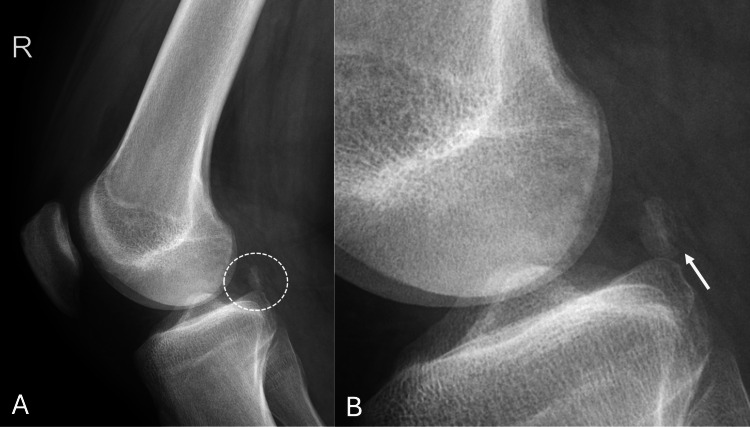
Follow up right knee X-ray (A) lateral view and (B) magnified image showing interval progressive healing of the right fabella fracture
